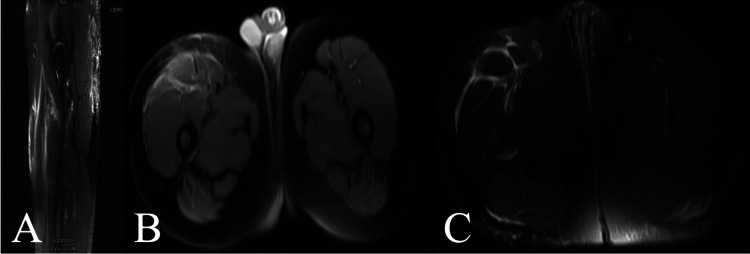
MRI showing patchy, edema-like signal throughout the right rectus femoris from the hip to the knee Frontal (A), transverse T1 (B), and transverse T2 (C) views
